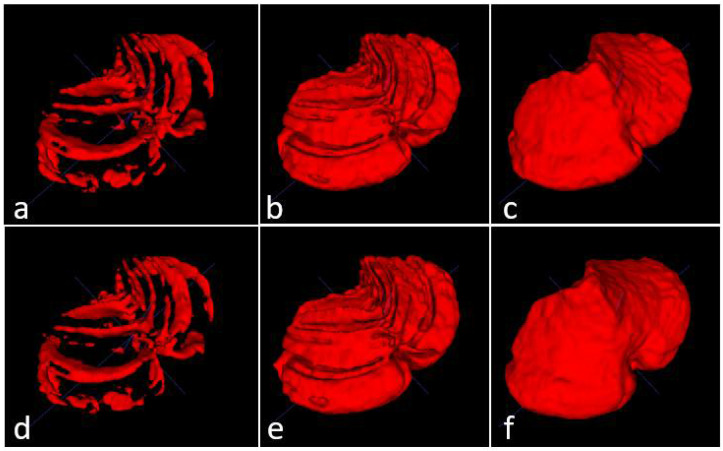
Masks and predictions for an MRI belonging to one of the SCA2 patients in the test subset. The top row shows the original masks, obtained with the procedure described in Section 2.2, and the bottom row displays the segmentations produced by our models. Cerebellar fissures in (a,d), cerebellum tissue with fissures in (b,e), and whole cerebellum without any fissure in (c,f).
